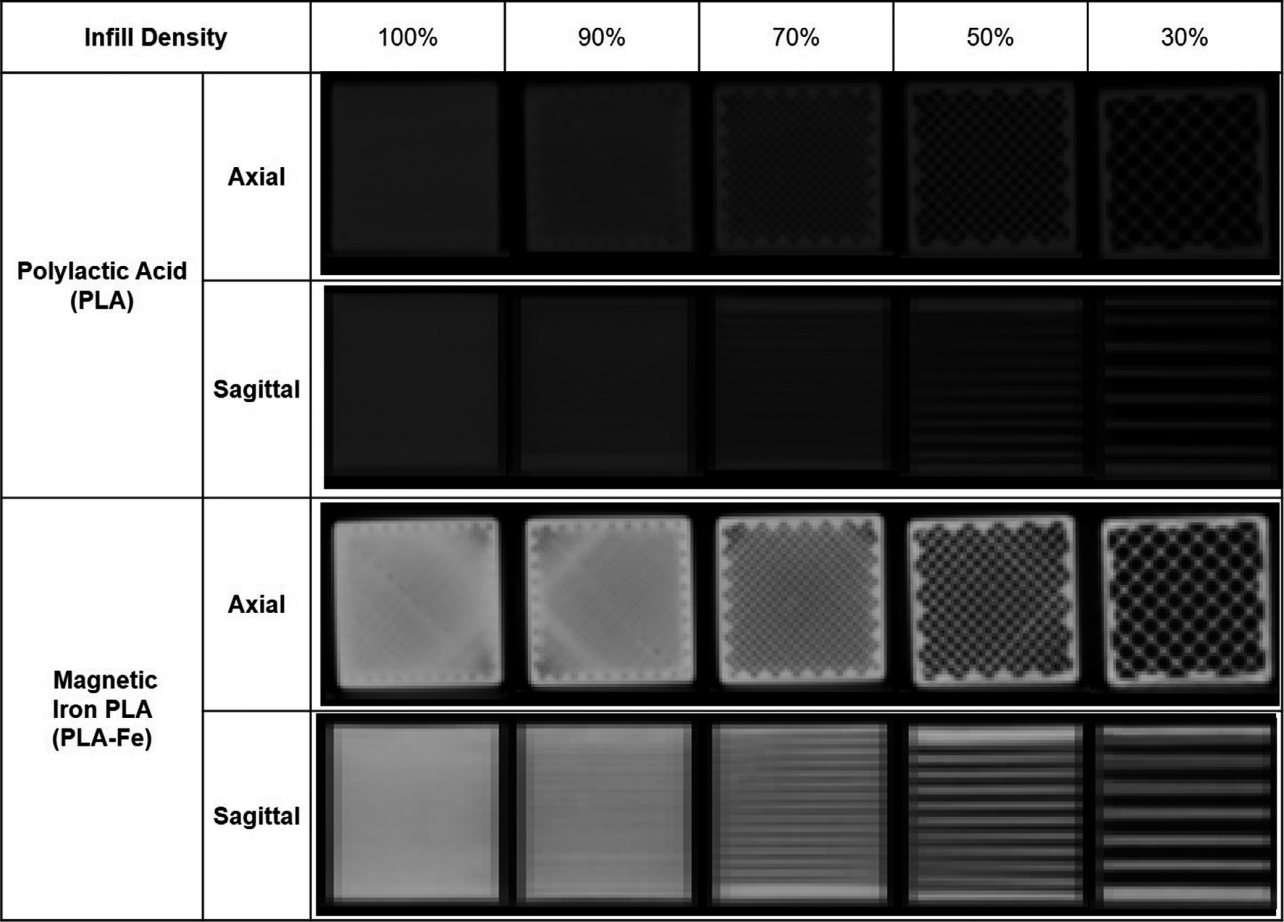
Sagittal and axial computed tomography images for the three‐dimensional ‐printed blocks using polylactic acid (PLA) and PLA‐Fe plastics with different infill densities.
